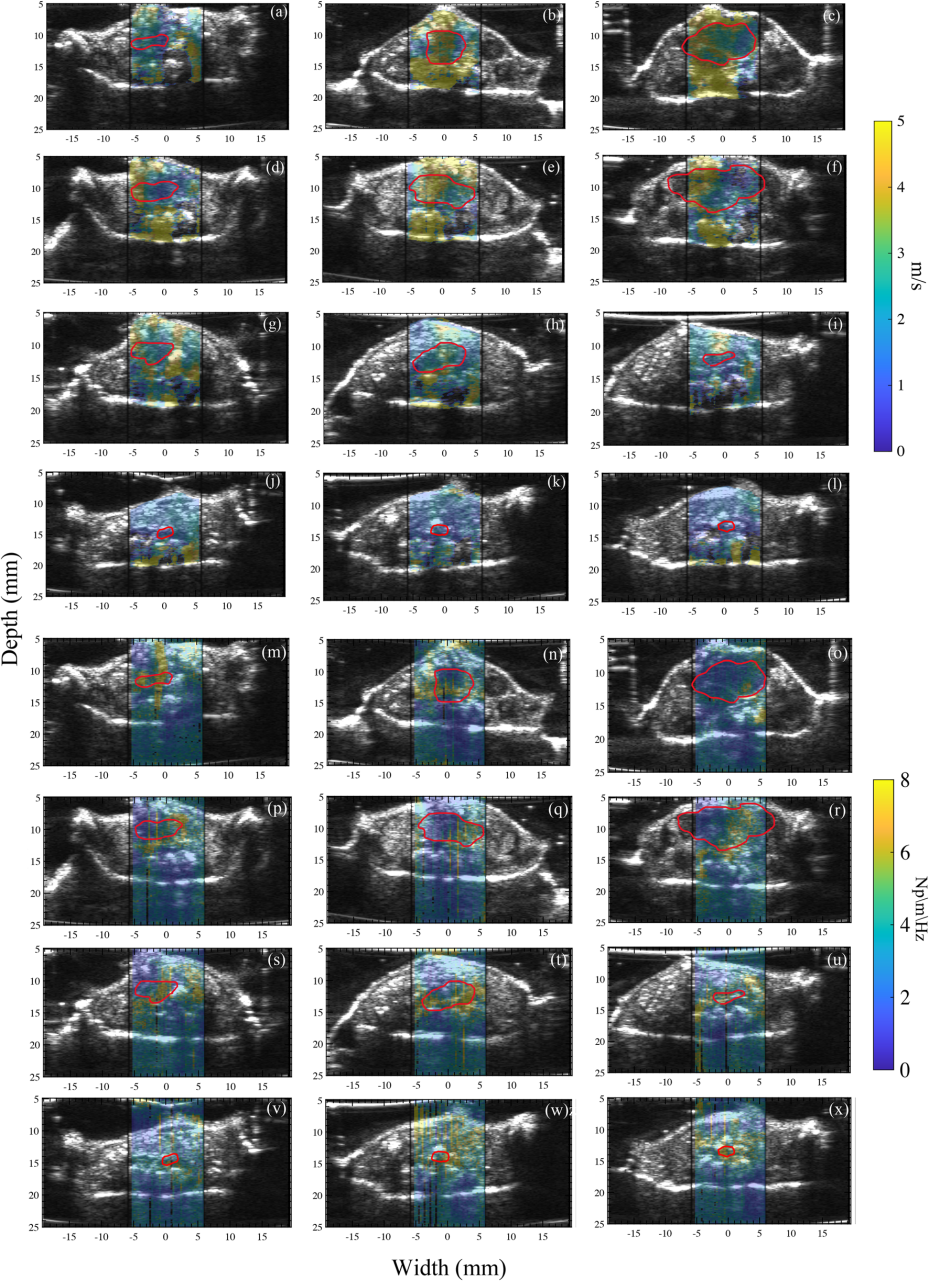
Sonograms displaying the shear wave speed (SWS, a–l) and shear wave attenuation (SWA, m–x) of representative samples where tumors are outlined in red. The samples include untreated tumors (a–c), tumors that were radiation-non-responsive to short-course radiotherapy (SCRT) (d–f), tumors that were responsive to SCRT (g–i), and healthy rectum tissue (j–l). The columns in the figure represent the SWS or SWA measurements taken on days 12, 16, and 20 post-injection (3, 7, and 11 post-SCRT). The sonograms with SWA are also shown for untreated (m–o), radiation-non-responsive tumors (p–r), radiation-responsive tumors (s–u), and healthy rectum (v–x). This figure provides a clear understanding of how SWS and SWA changes in different tissue types over time compared to healthy rectum tissue.
